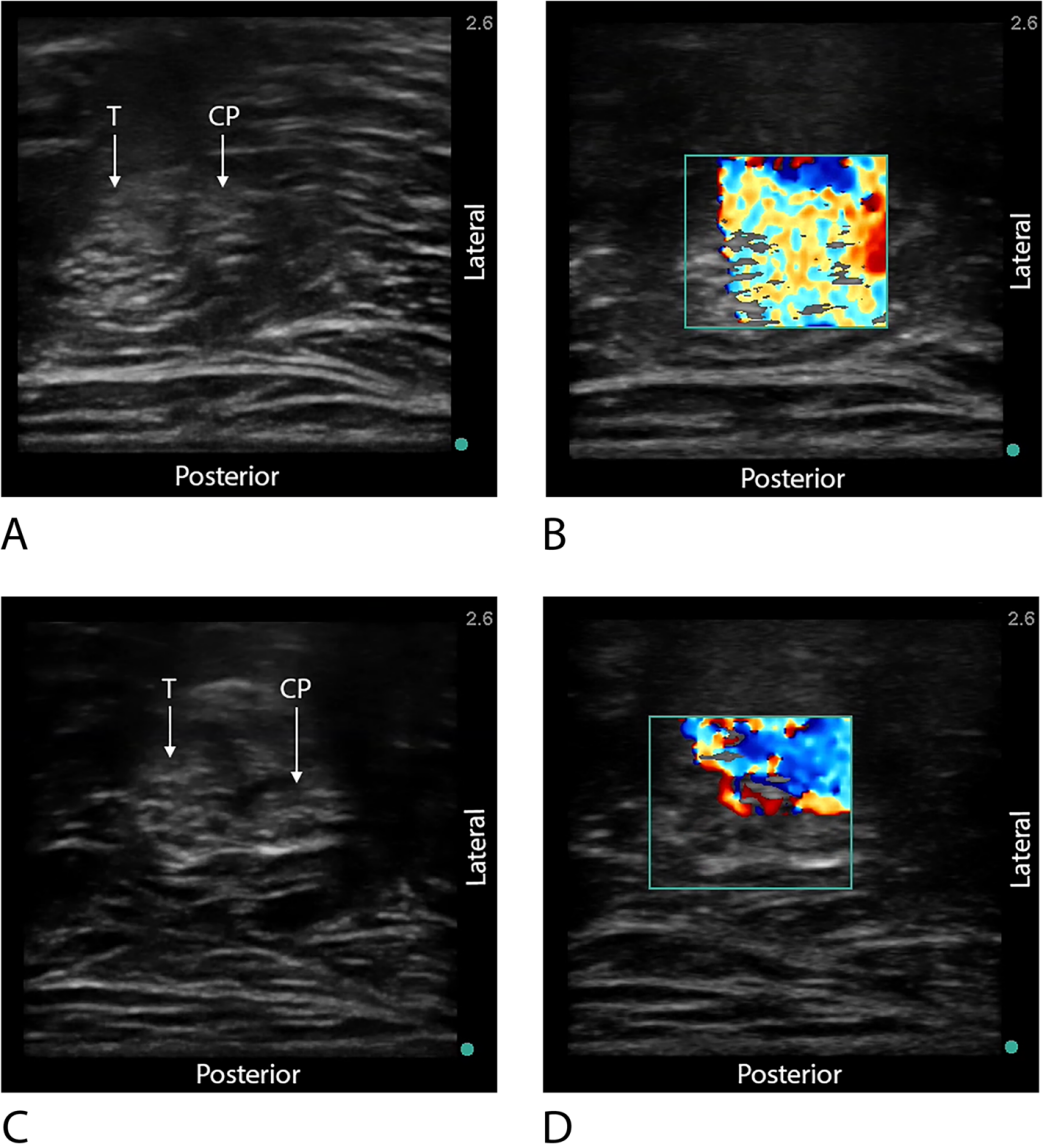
A, C After perineural catheter placement, ultrasound images displaying bifurcation of the sciatic nerve into tibial and common peroneal branches. Note, the catheter is not seen. B Doppler ultrasound image demonstrating complete sciatic nerve coverage, ≥ 50% of tibial and common peroneal branches are covered by the agitation solution. D Doppler ultrasound image demonstrating partial coverage of the sciatic nerve, < 50% of the tibial and common peroneal branches are covered by the agitation solution. T, tibial branch; CP, common peroneal branch
